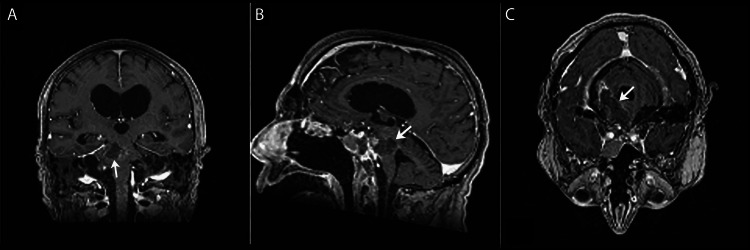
Postoperative MRI T1 scans in coronal (A), sagittal (B), and axial (C) views. These post-operative T1-weighted MRI scans confirm the absence of residual chordoma.
